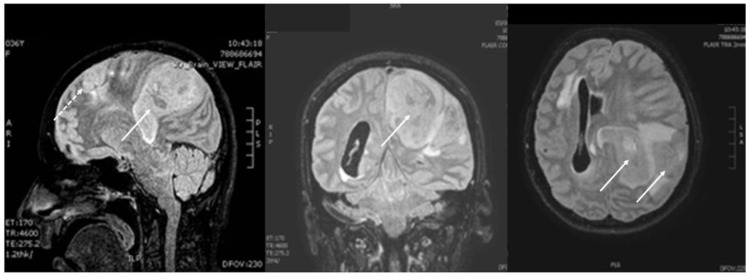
Brain MRI (FLAIR images) upon the second admission to ICU due to neurological deterioration: recurrence of the primary tumor in the right parietal lobe (marked with dotted white arrow) and a large new mass in the same lobe (marked with solid white arrow), deforming the right lateral ventricle down to the brain stem.
